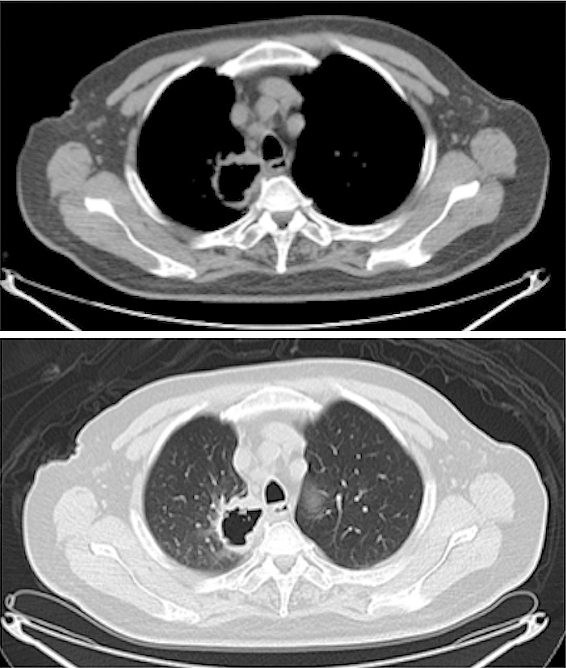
Computed tomography (CT) image reveals a mass with a thick-walled cavity in the right upper lobe of the lung.
